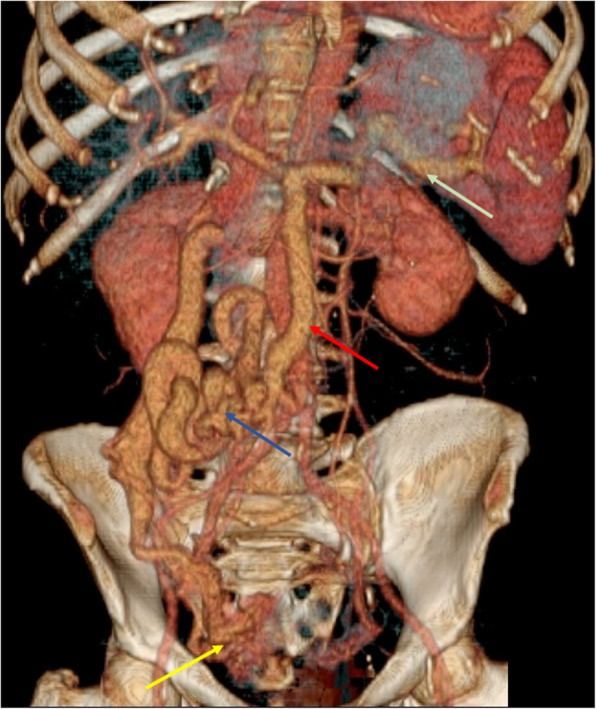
Retroperitoneal varices. VRT image from portal venous phase CT shows an increase in size of SV (green arrow) and SMV (red arrow), an extensive collateral network of varices fed by SMV (blue arrows), communicating with a huge-caliber right ovarian vein (yellow arrow), and giving rise to varices along the ovarian venous plexus
